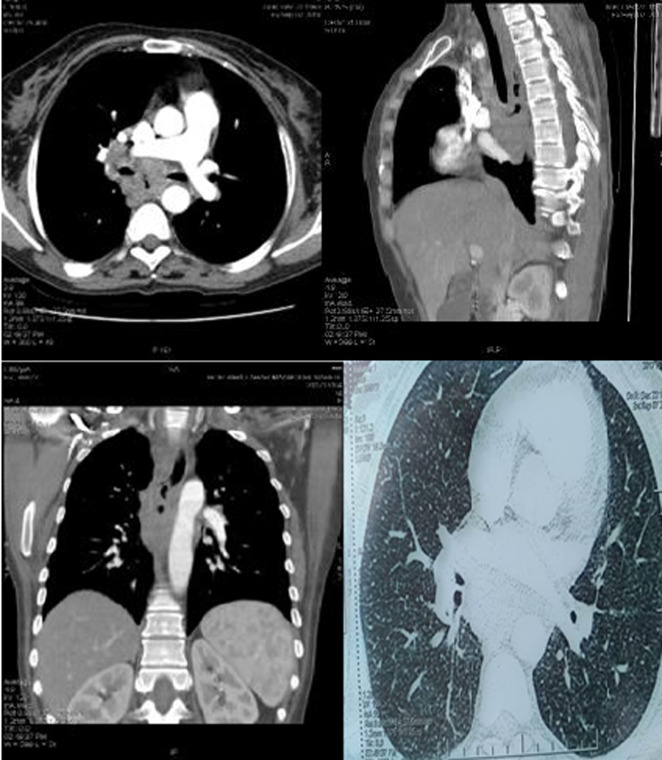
CT-scan findings: esophago-mediastinal fistula, mediastinal adenopathies and military tuberculosis
